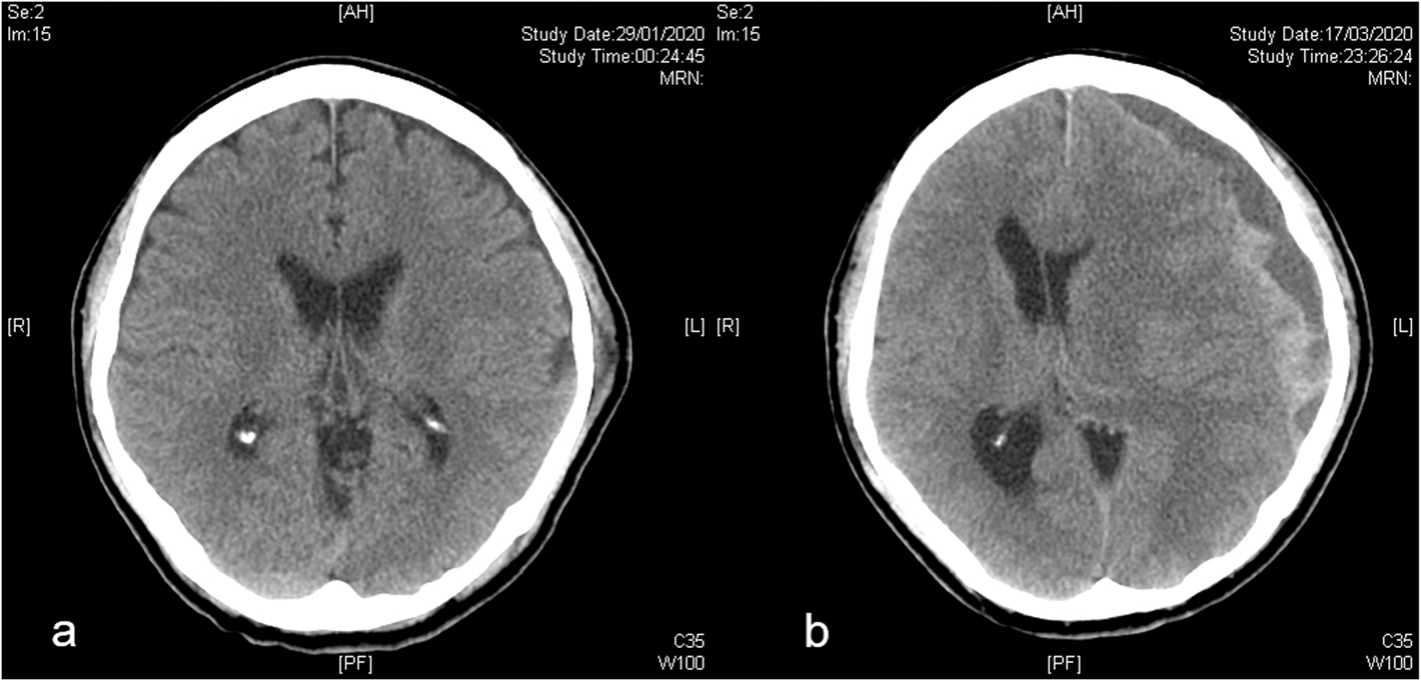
a Computed tomographic (CT) scan of the brain of a 59-year-old gentleman at the time of injury. He had a minor head injury with retrograde amnesia. CT scan showed a left-sided scalp hematoma but no intracranial hemorrhage and he was discharged with medical advice. Subsequently, he had a progressively worsening headache. b He finally re-attended the hospital 7 weeks after the initial minor head injury. The new CT showed a left-sided chronic subdural hematoma with mass effect and midline shift. He had a good recovery after emergency burr hole drainage
